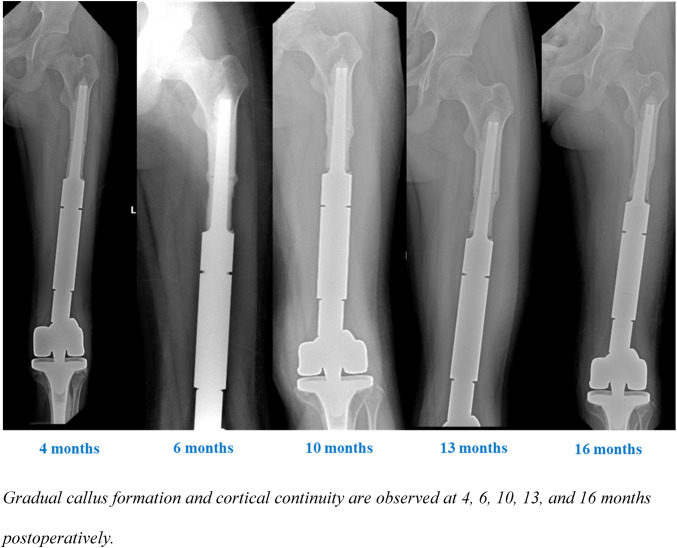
Serial radiographs demonstrating progressive bone union after reconstruction using a liquid nitrogen-treated autograft combined with a megaprosthesis
